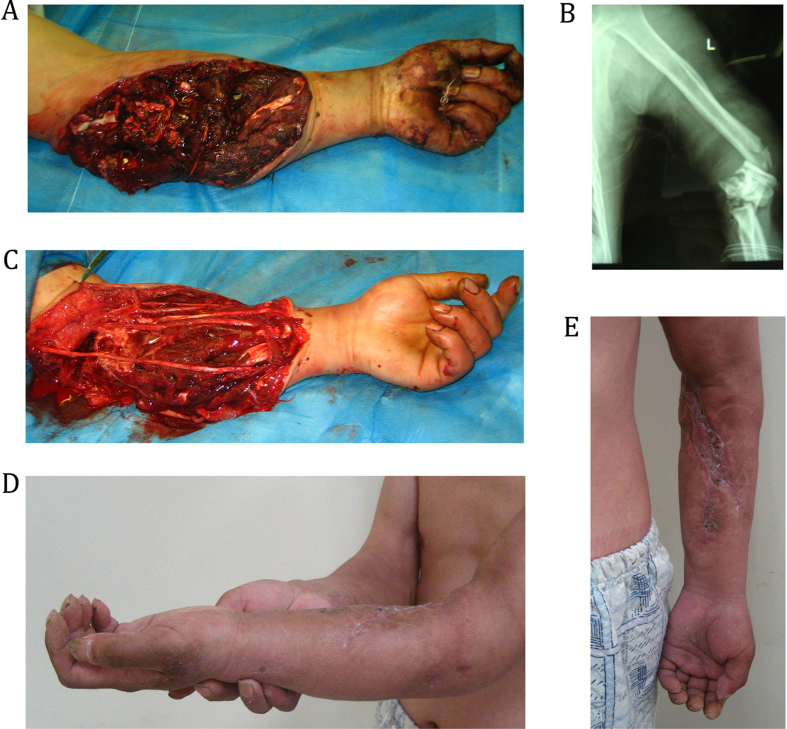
A 45-year-old man sustained a crush injury in an industrial accident and was referred to our institute after 8 h had elapsed. (A) Preoperative detection found that Ulnar and radial arteriovenous ruptured. (B) X-ray show the fracture in distal humerus and elbow joint. (C) End-to-end anastomoses to rebuild the vasculature. (D,E) The limb was successfully salvaged eventually and the patient had achieved 84 points when he was discharged.
